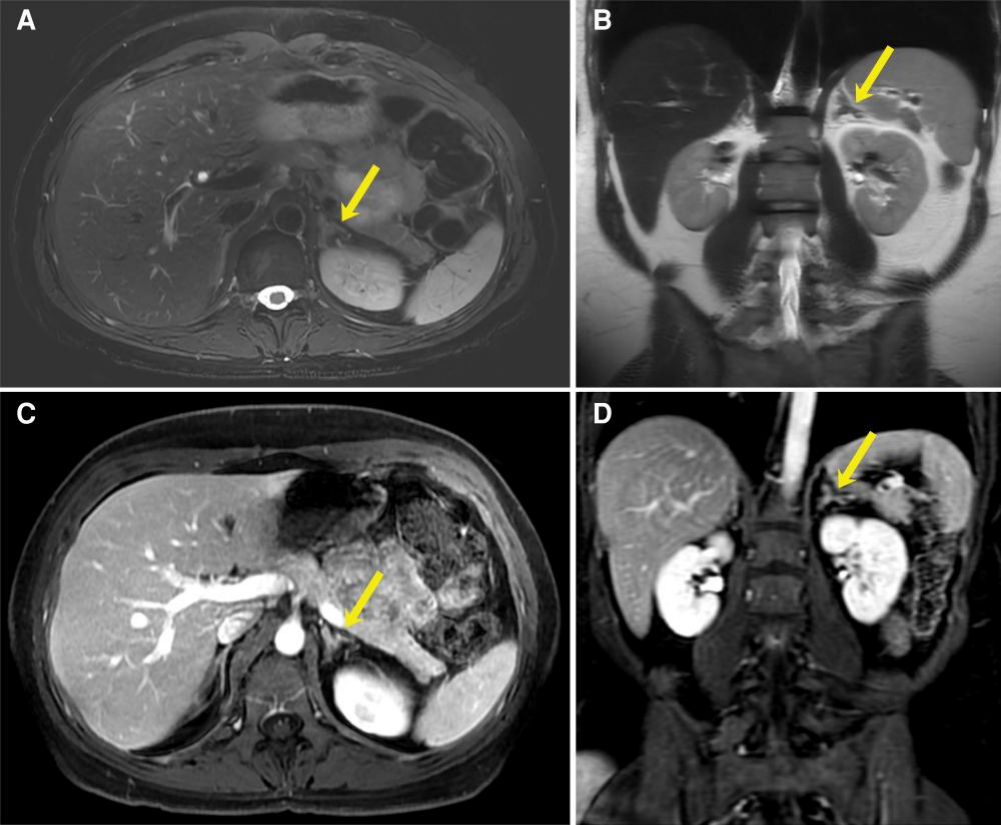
Diagnostic MRI scan showing complete resolution of the left adrenal cyst 7 months following percutaneous aspiration and sclerotherapy. (A) Axial T2-weighted fat-saturated, (B) coronal T2-weighted nonfat-saturated, and (C) axial and (D) coronal T1-weighted postgadolinium-enhanced MRI demonstrates morphologically normal appearing left adrenal gland (arrow) with no residual cyst.
